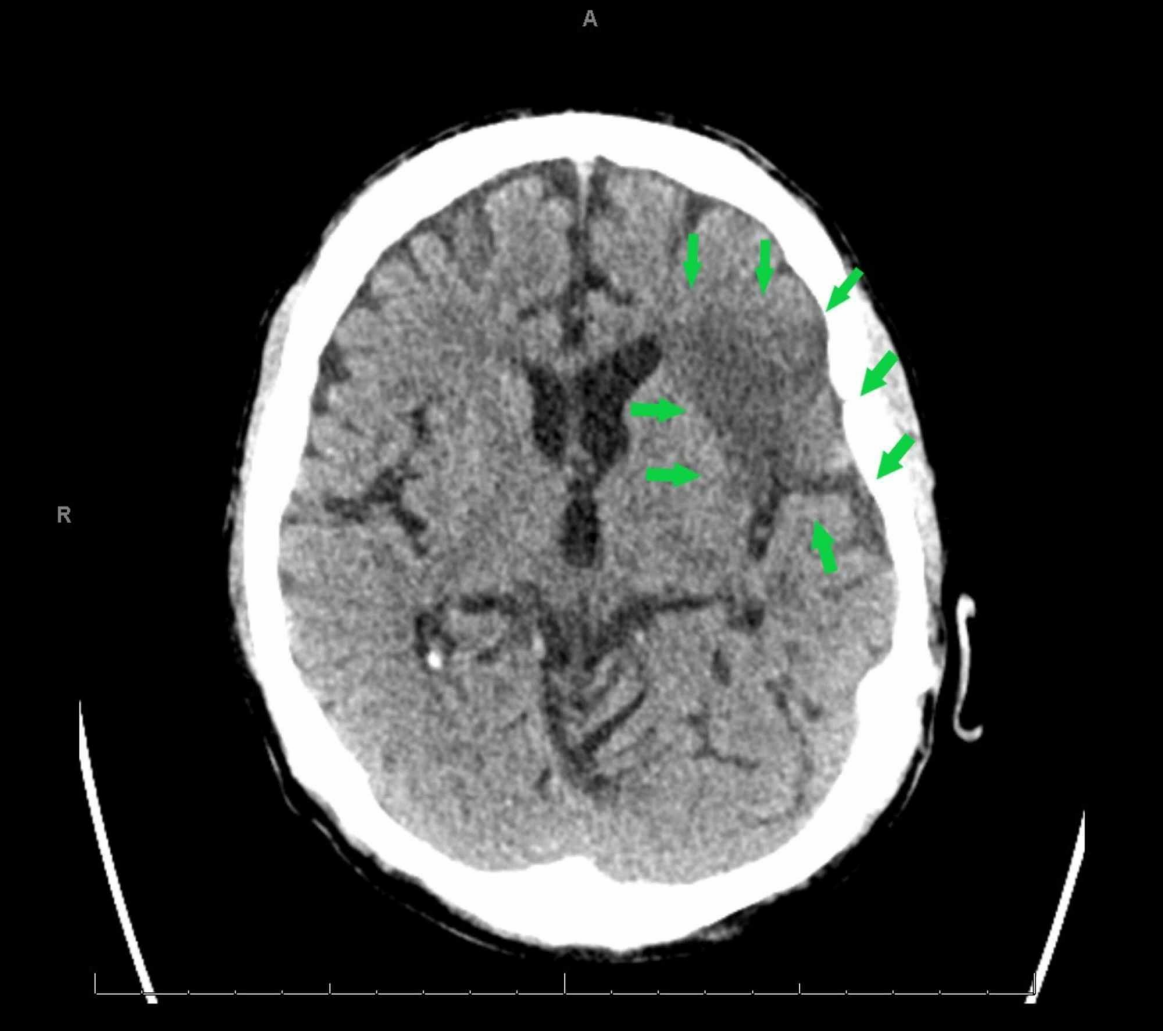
Computed tomography (CT) of the head without contrast revealing an acute left frontal infarct (arrows) 24 hours after admission
